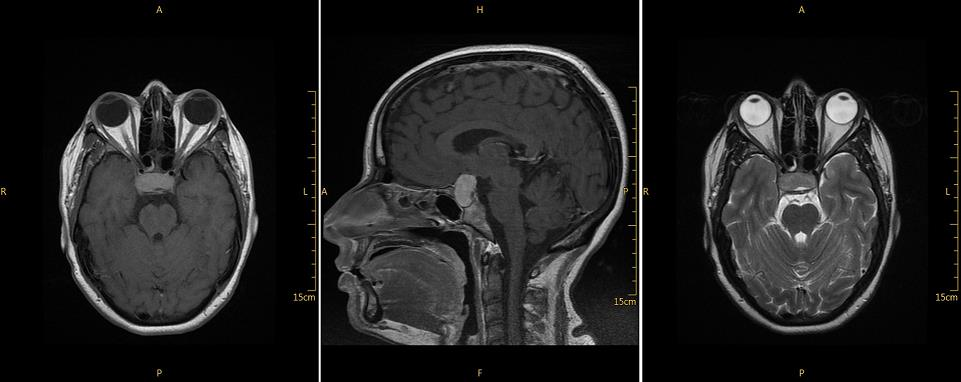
MRI revealed large sellar mass with lateral and supra sellar extension compressed optic chiasma and intense homogenous enhancement
